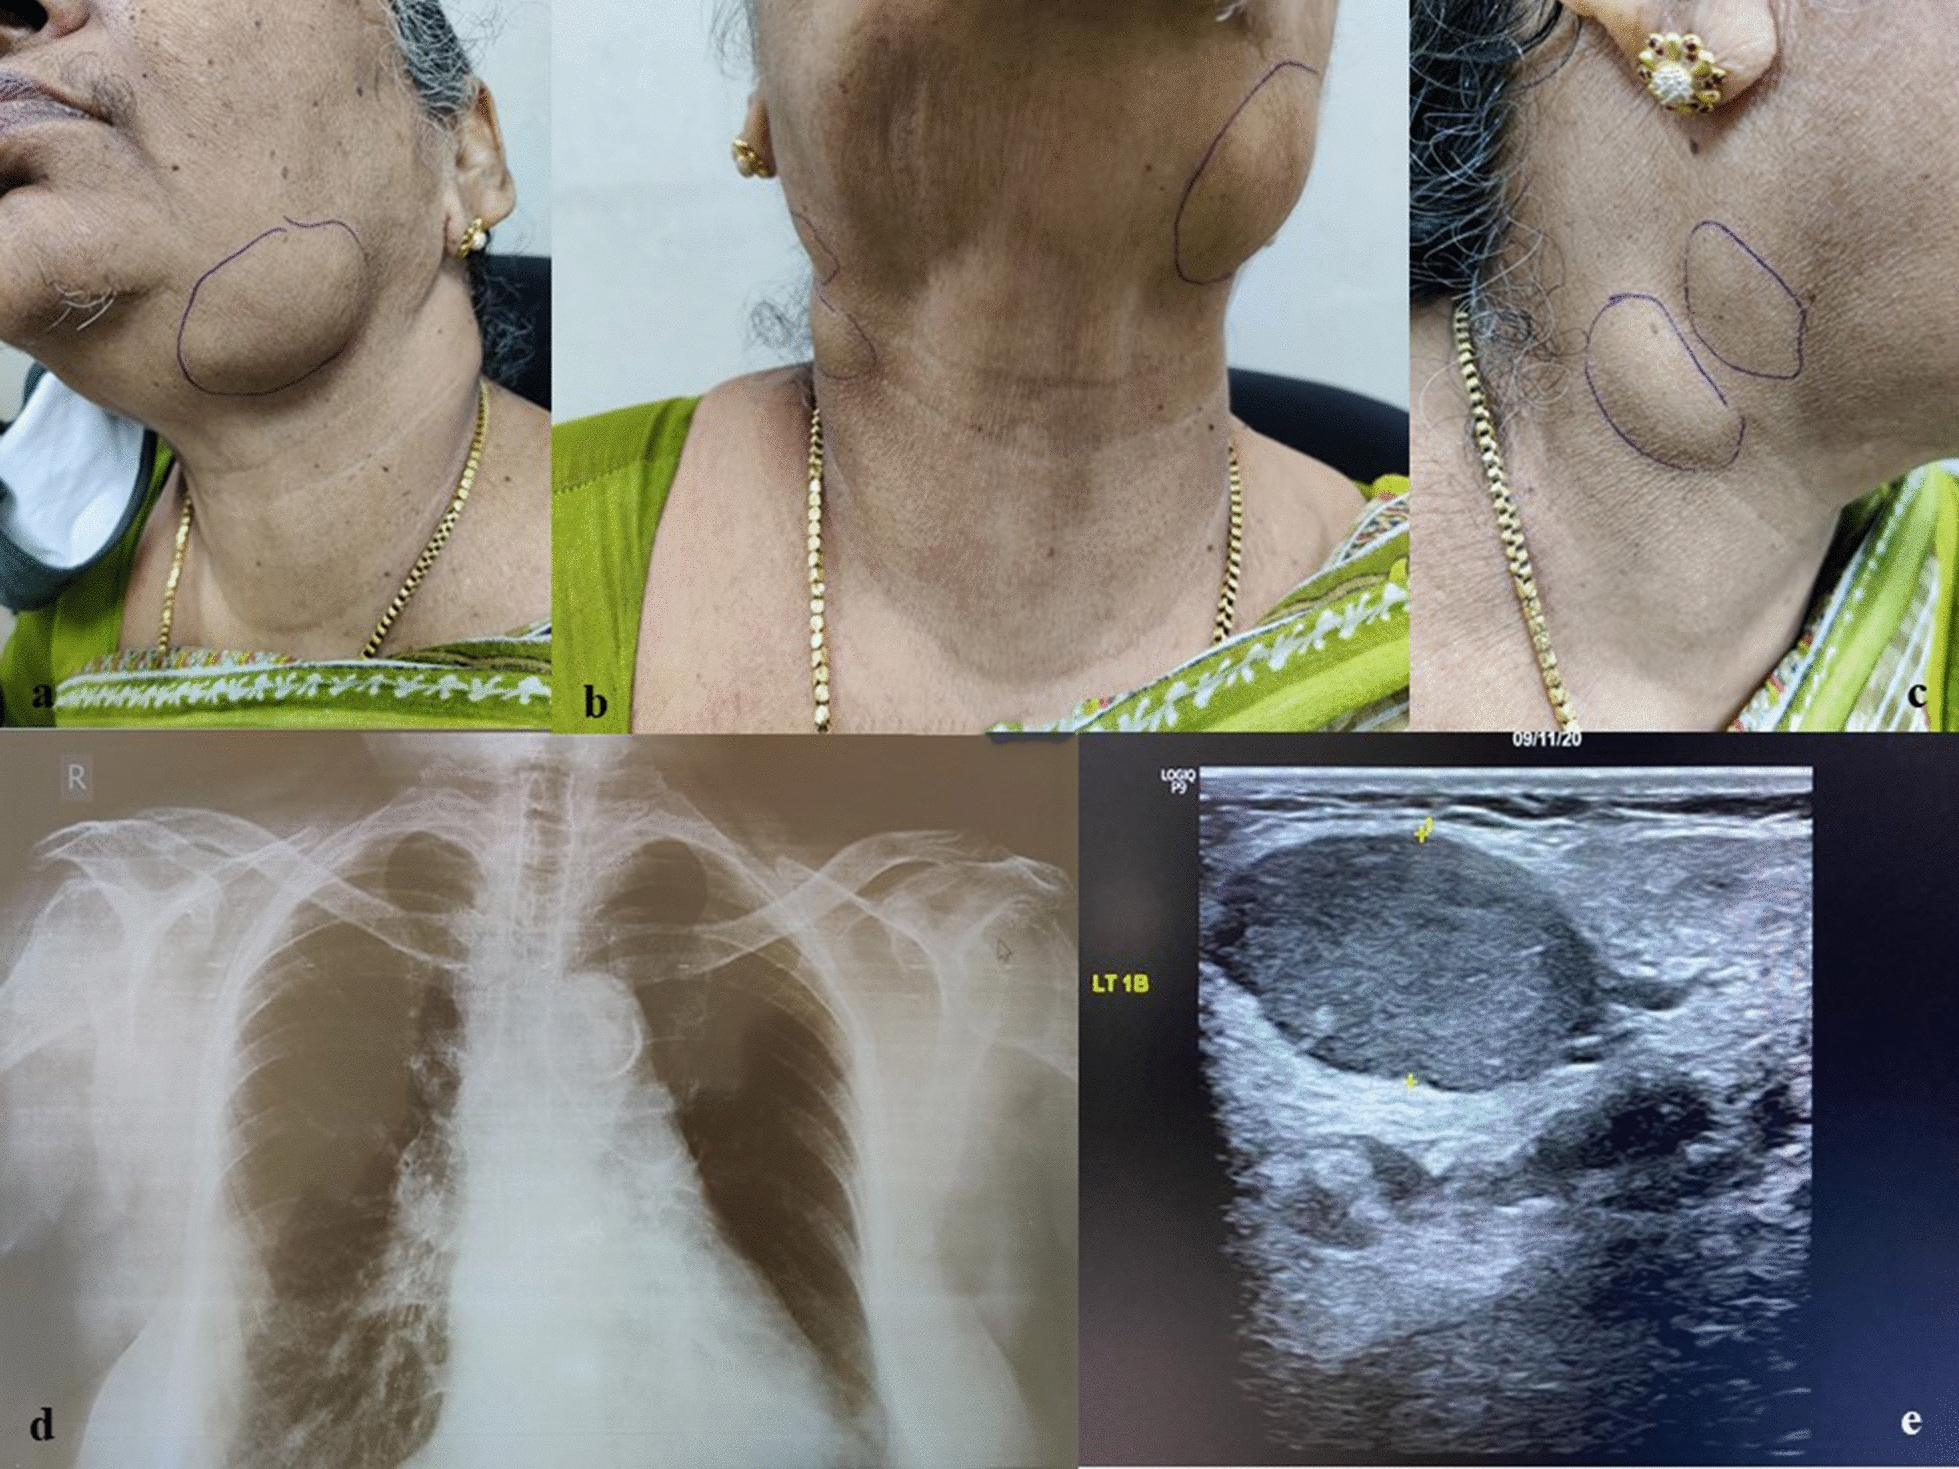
a–c Patient with multiple matted lymph nodes on left side level Ib, right level IIb, III, and grade I thyroid swelling. d Chest X-ray showing bilateral prominent hilar shadow with clear bilateral lung fields. e USG neck with level 1b lymph node on left side, hypoechoic and round measuring 2.4 × 1.0 cm
